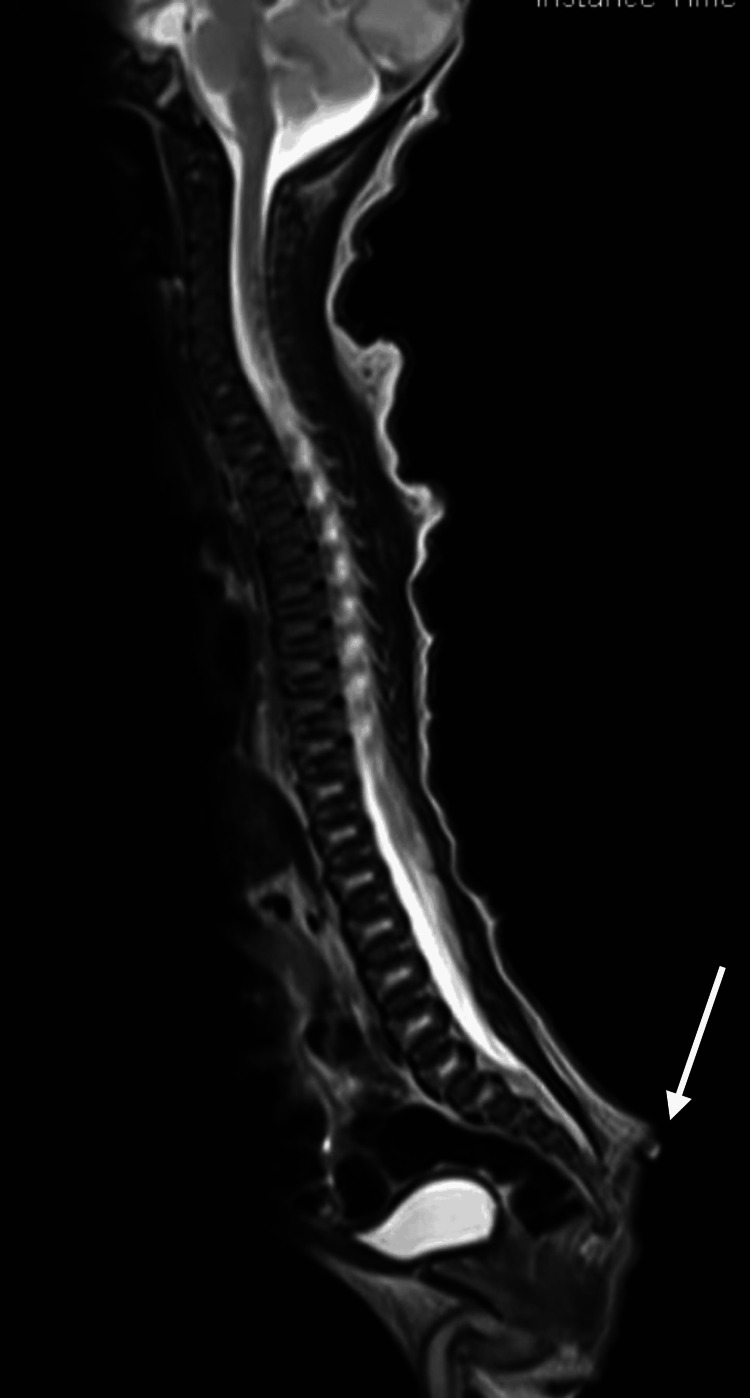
MRI of the spine shows a pedunculated appendage with fat intensity hanging from the mid-sacral region (arrow)
